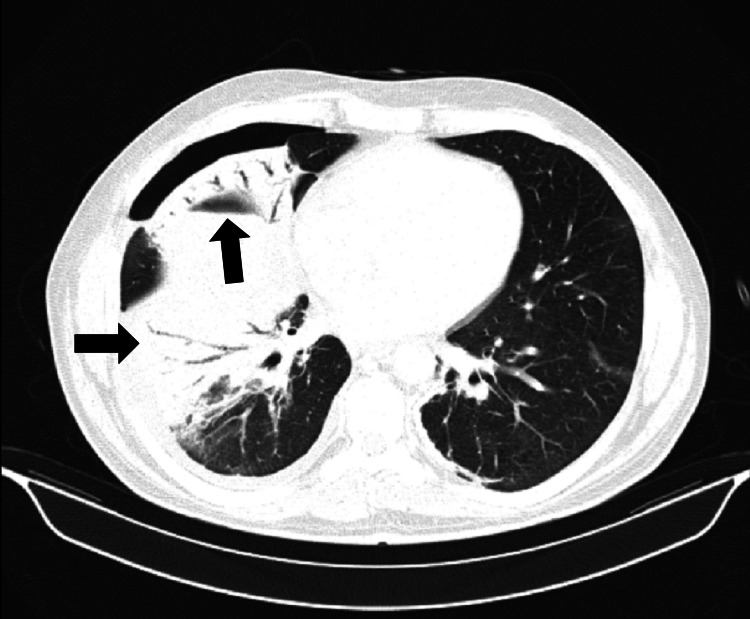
Chest CT on the 8th day of hospitalization, axial view. Chest CT on the 8th day of hospitalization, corresponding to the patient's clinical deterioration, showing new consolidation in the right lung with an air bronchogram with loss of volume at the middle lobe.
